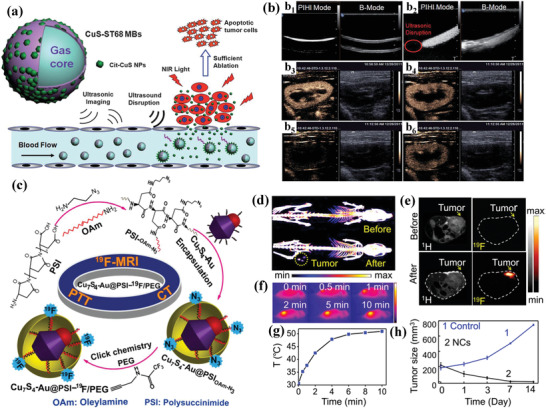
a) The scheme of the theranostic functionality of CuS‐ST68 MBs for ultrasound imaging and UTMD‐induced photothermal ablation. b) In vitro ultrasound imaging in a latex tube b_1_) in the presence and b_2_) in the absence of CuS‐ST68 MBs under PIHI mode and B mode. In vivo ultrasound imaging of rabbit right kidney after the administration of b_3_) ST68 MBs and b_4_) CuS‐ST68 MBs. b_5_) Ultrasound imaging of rabbit right kidney after focused ultrasonic disruption of CuS‐ST68 MBs and b_6_) reperfusion of CuS‐ST68 MBs into kidneys for ultrasound imaging. Reproduced with permission.^[^
